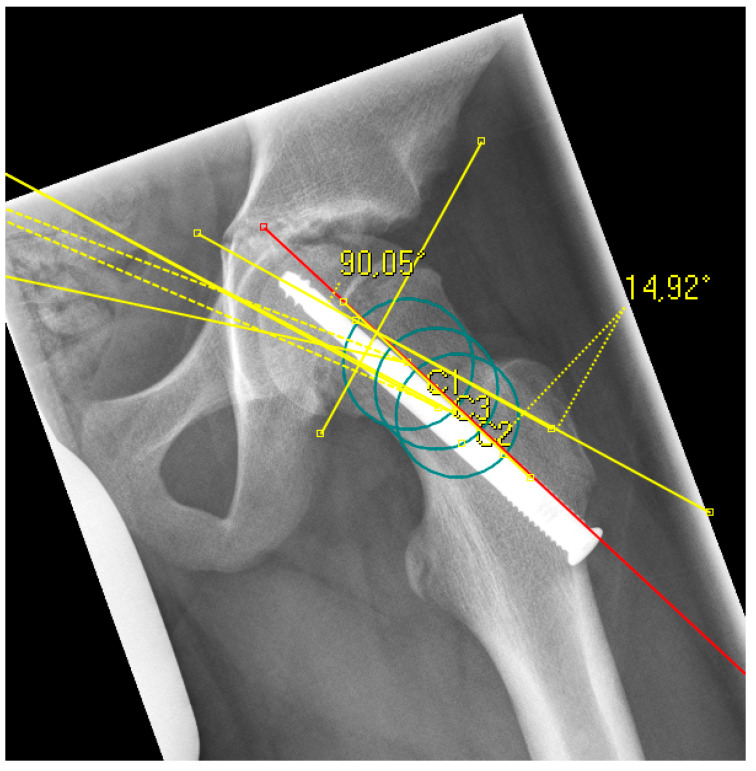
Example of a radiograph with the measurement of the epiphyseal tilt based on the anatomical femoral neck axis (red line), which was determined with three circles (green) according to Bouma [29].
